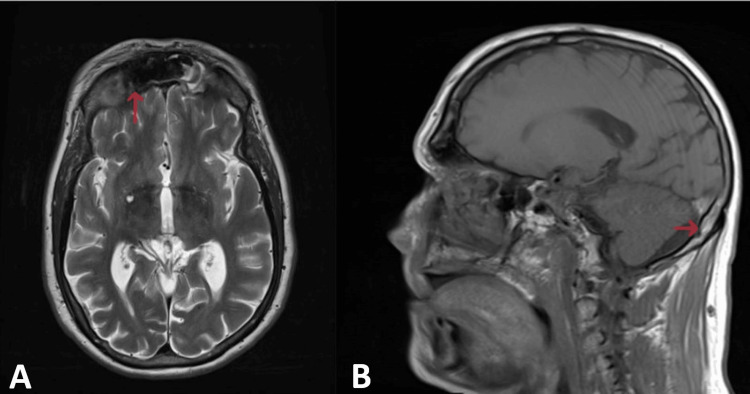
MRI of the brain with and without IV contrast MRI of the brain with and without IV contrast showed acute-on-chronic pansinusitis with associated frontal bone osteomyelitis (A: red arrow on axial view) and subperiosteal phlegmon suggestive of Pott's puffy tumor with complications of pachymeningitis (B: red arrows on sagittal view). Additional findings of periorbital cellulitis were also found. No cerebritis, brain abscess, acute infarct, or hemorrhage was noted.
